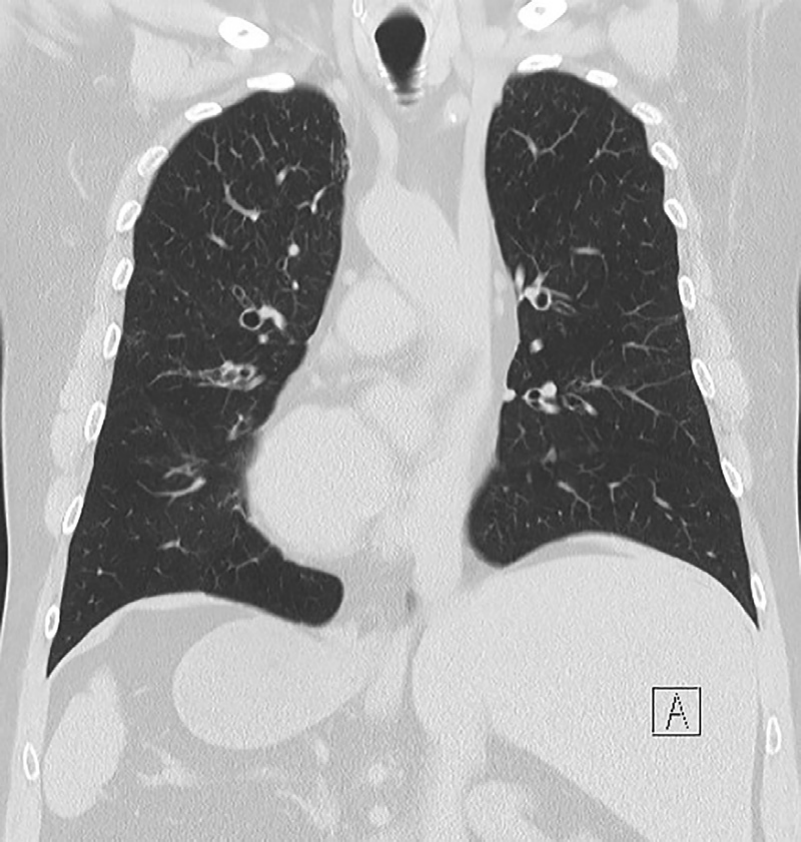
A coronal section of a high-resolution chest CT scan demonstrating bronchiectasis and dextrocardia with situs invertus totalis in an individual with PCD.
